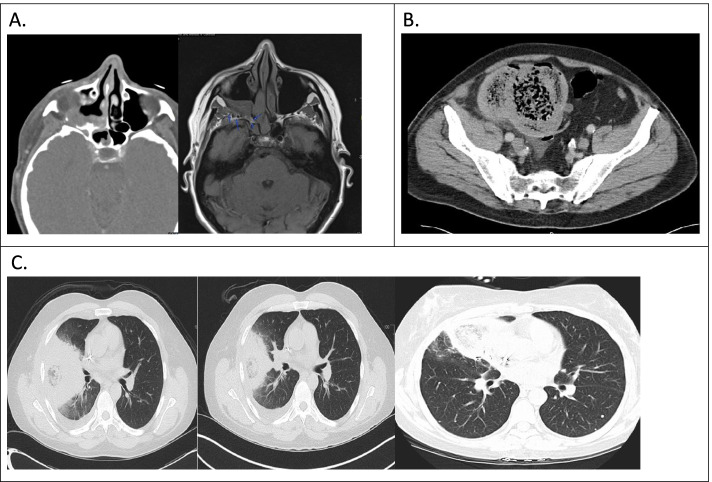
Radiographic signs of mucormycosis. A Maxillary sinus mucormycosis. B Gastrointestinal mucormycosis of cecum. C Pulmonary mucormycosis: reversed halo sign on CT, an area of ground glass opacity surrounded by a ring of consolidation
